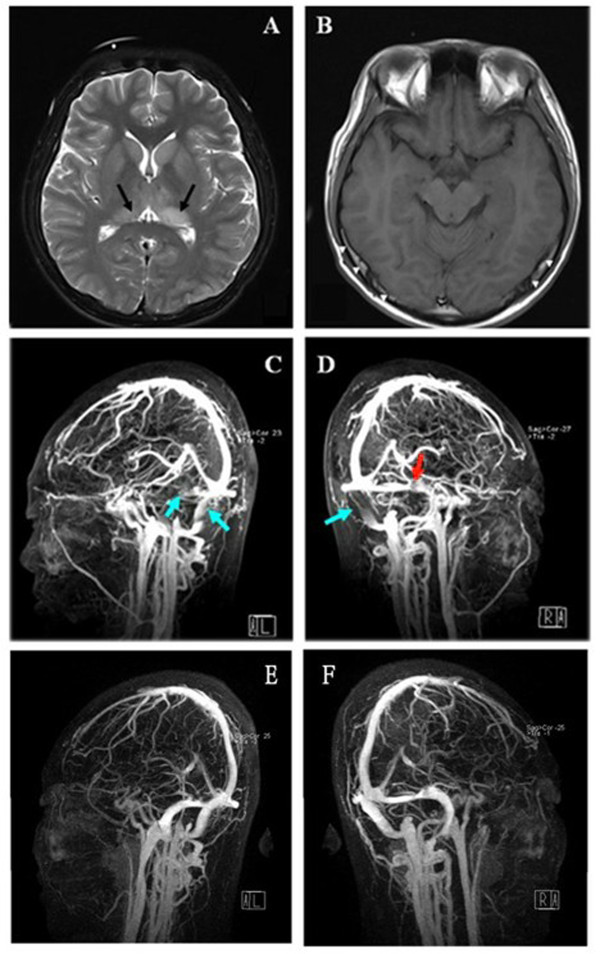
MR imaging of the patient before and after treatment. (A) Axial T2-weighted images showed the swelling of bilateral thalamus. (B) Contrast Enhanced MRI scan revealed sigmoid sinus and transverse sinus filling defect (white arrows), indicating venous thrombosis. (C and D) The MRV scan indicated absence of signal in the bilateral sigmoid sinuses and left transverse sinuses before treatment (blue arrows). (D) Red arrow showed an arachnoid granulation not venous thrombosis in the right transverse sinus. (E, F) The repeat MRV scan demonstrated only a few low signal areas in the left sigmoid sinus after treatment.
